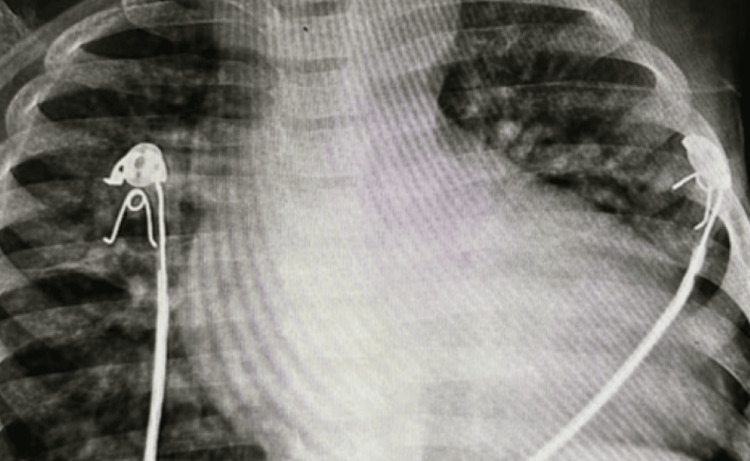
Chest X-ray PA view showing minimally oligemic lung field with the cardiac shadow showing an “egg on string” appearance PA: Posteroanterior
